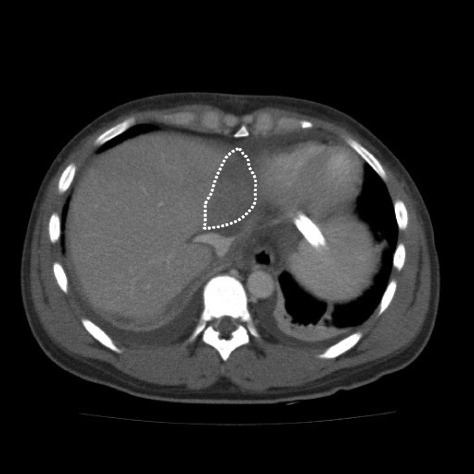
Computed tomographic images on POD 6 showing necrosis of segment IV of the transplanted graft
